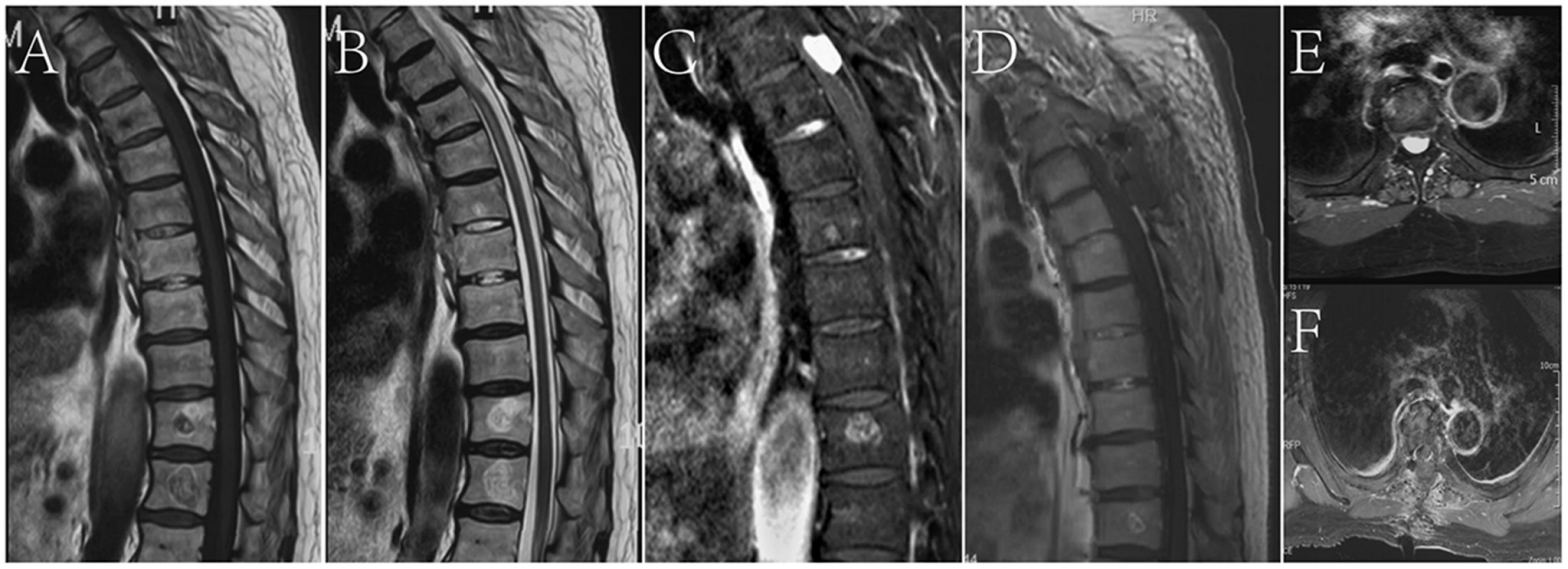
(A,B) The tumor presented isosignals on T1WI and T2WI. (C,E) The mass showed uniform enhancement after contrast agent injection. (D,F) Post-operative review by thoracic MRI showed that all tumors had been removed.
